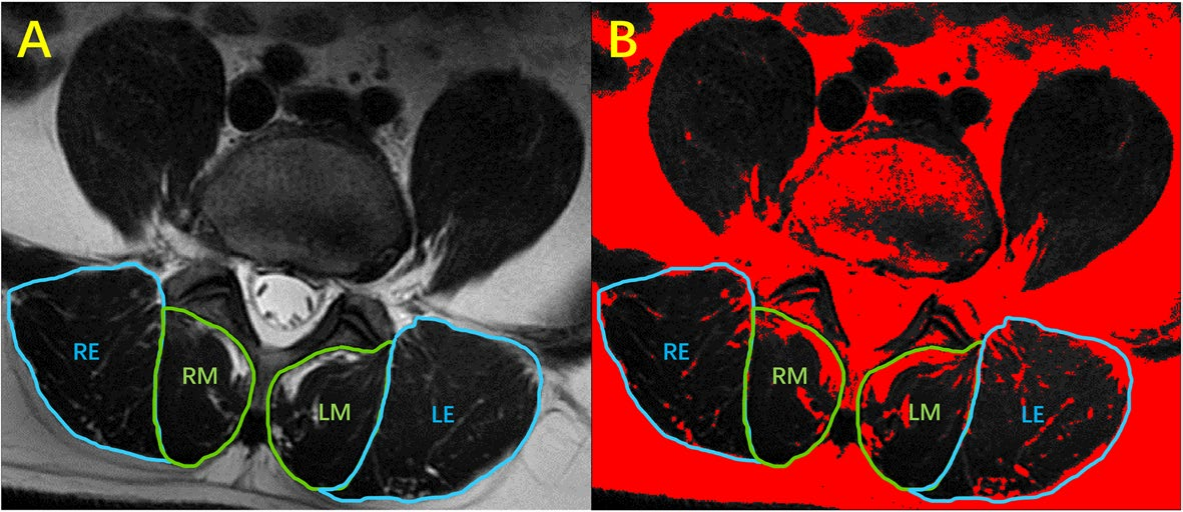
Fig. 1.A is a T2-weighted image of the axial L4-L5 disc level. RE was the CSA (cross-sectional area) of the right erector spinae, LE was the CSA of the left erector spinae, RM was the CSA of the right multifidus, LM was the CSA of the left multifidus. Figure 1.B is the image obtained from threshold processing of A by Image J. Fat (red area) and muscle tissue (without highlight area) were segmented, and the red area can be calculated by the software. tCSA = RE + RM + LE + LM, fCSA = tCSA-fat tissue (read area in four circles)
